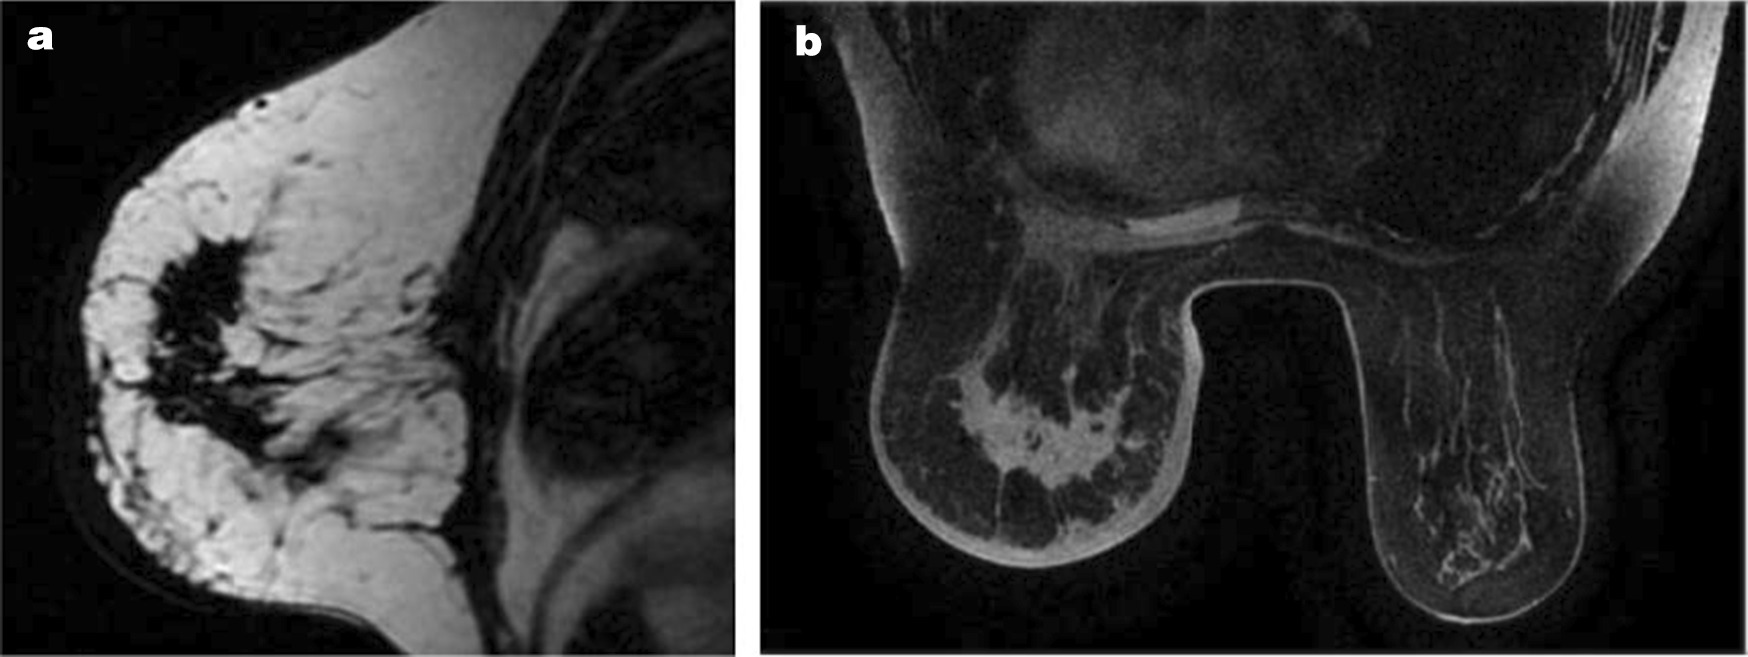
Magnetic resonance imaging (MRI). MRI showed the heterogeneously distributed lesion in an area of high signal intensity in the left breast on T1-weighted images. The breast mass was rapidly enhanced following the injection of gadolinium diethylenetriamine-pentaacetic acid at an early phase (2 minutes after agent injection).
